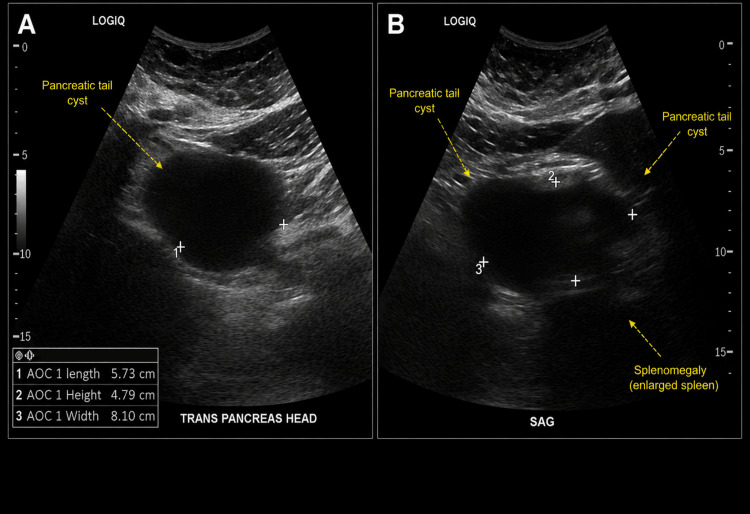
Abdominal ultrasound demonstrating interval enlargement of the pancreatic tail cyst. (A) Transverse ultrasound view demonstrating a large cystic lesion arising from the pancreatic tail with measured dimensions of 8.10 cm × 5.73 cm × 4.79 cm. (B) Sagittal ultrasound view confirming the cystic pancreatic tail lesion.
